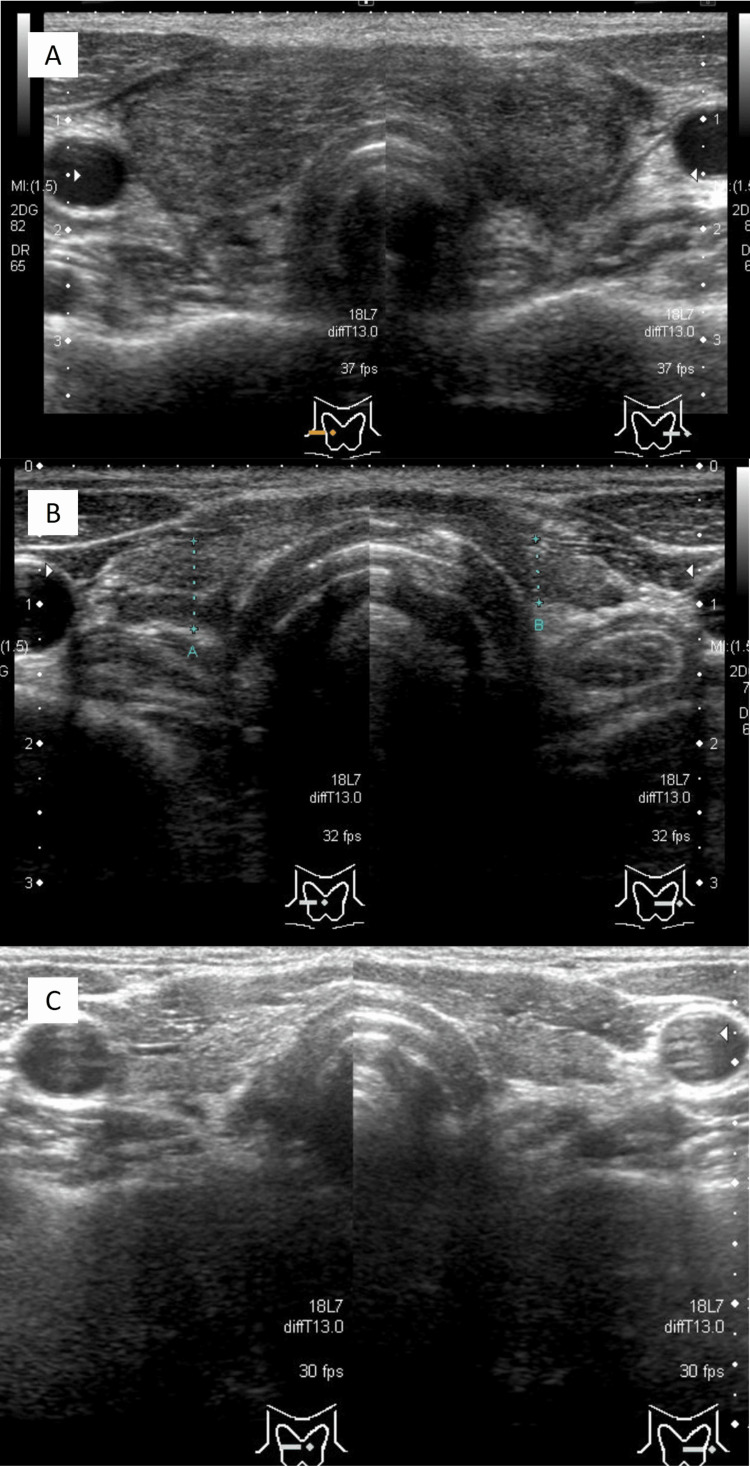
Thyroid ultrasound findings of Patient two. A:Day +99(at onset), right lobe 19 mm, isthmus 4 mm, left lobe 17 mm B:Day +293(at follow up), right lobe 6 mm, isthmus 1 mm, left lobe 5 mm C:Day +1076(at follow up), right lobe 5 mm, isthmus 1 mm, left lobe 5 mm
